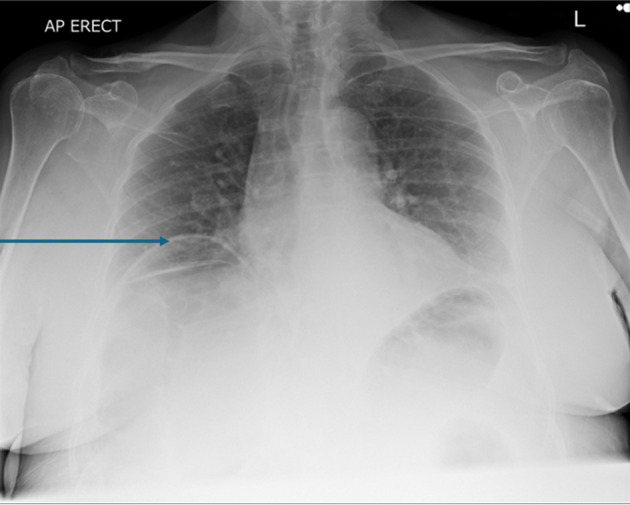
Anterior-posterior chest X-ray on the day of symptoms beginning showing bilateral gas filled loops beneath both hemidiaphragms more in the right side. The arrow points towards elevated right hemidiaphragm. Images taken day 1 postoperatively.
